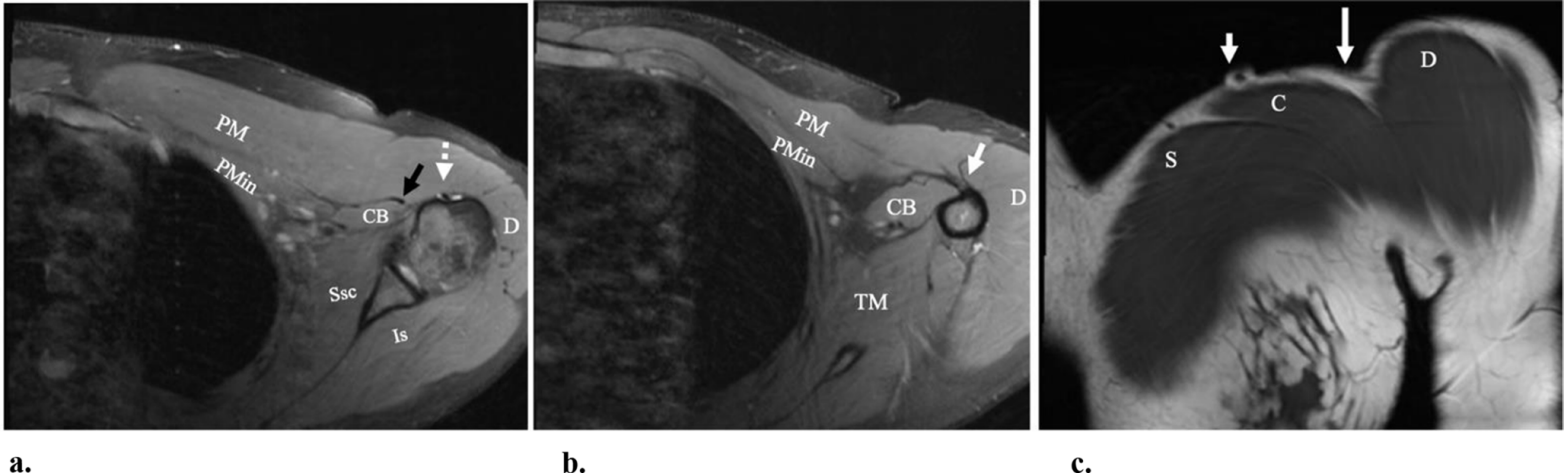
Normal anatomy in a 21-year-old-female that presented with pain in the clavicular region, with the marker (short arrow in c) overlying the site of pain. (a) and (b) Axial proton-density-weighted fat-saturated MR image shows the pectoralis major muscle (PM) at two levels, with (a) above the level of (b). More cranially in (a), the normal position and appearance of the long head of the biceps tendon (dashed arrow in a) in the bicipital groove is denoted. Also note the tendons of the coracobrachialis and short head of the biceps (black arrow in a). The pectoralis minor muscle (PMin), coracobrachialis muscle (CB), subscapularis muscle (Ssc), infraspinatus muscle (Is) and the deltoid muscle (D) are also shown. More caudally in (b), the PM tendon as it approaches the humeral insertion (arrow in b) at the lateral lip of the bicipital groove is shown. The teres major muscle (TM) is shown, in addition to the other abbreviated muscle from (a). (c) Coronal T1-weighted MR image shows the clavicular (C) and sternal (S) heads of the pectoralis major muscle. Note the deltopectoral groove (long arrow in c), which separates the deltoid muscle (D) from the head of the pectoralis major muscle. The obliquely oriented tubular low signal in the subcutaneous fat in this groove, subjacent to the arrow, is the cephalic vein
